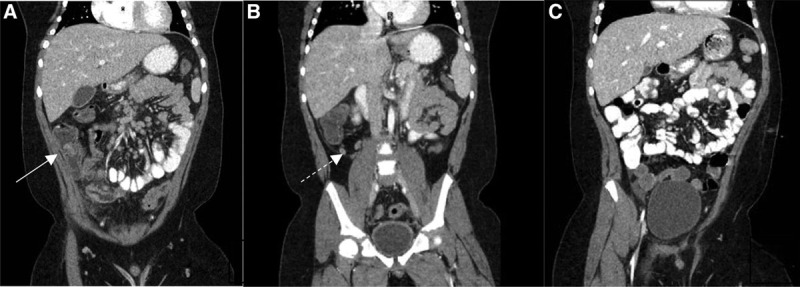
CT scan with oral and intravenous contrast of the abdomen and pelvis. A and B, CT scan of the abdomen and pelvis on presentation showing extensive phlegmonous changes in the right lower quadrant of the abdomen around the ileocecal junction (white arrowhead) with intact appendiceal wall (dashed arrowhead). C, CT scan of the abdomen and pelvis demonstrating complete resolution of phlegmonous ileocolitis after treatment. CT = computed tomography.
